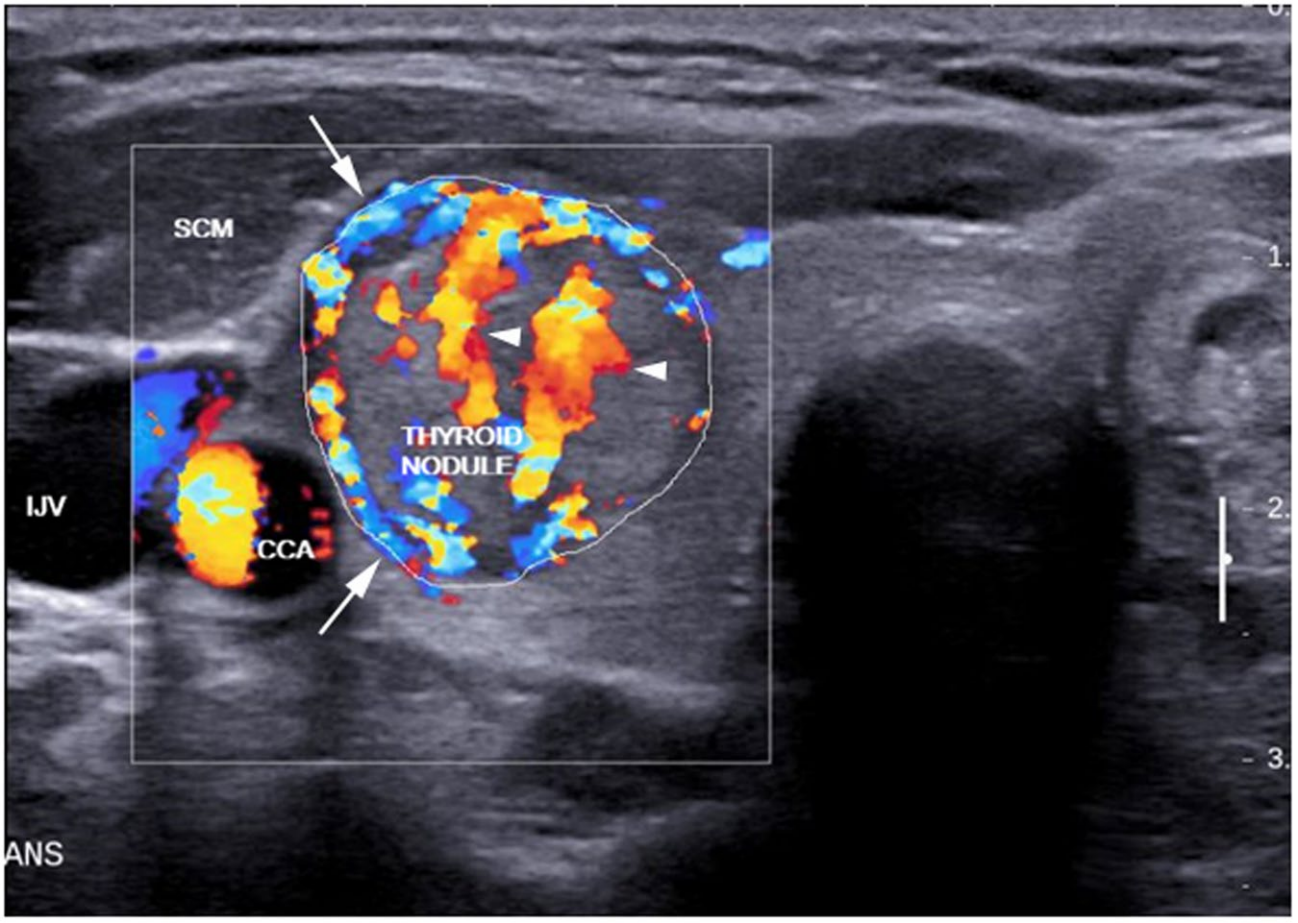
Transverse color Doppler sonogram of a nodule in the right thyroid lobe which was proven to be follicular thyroid carcinoma (outlined). The nodule showed both peripheral (arrows) and central (arrowheads) vascularity. SCM, sternocleidomastoid muscle; CCA, common carotid artery; IJV, internal jugular vein.
